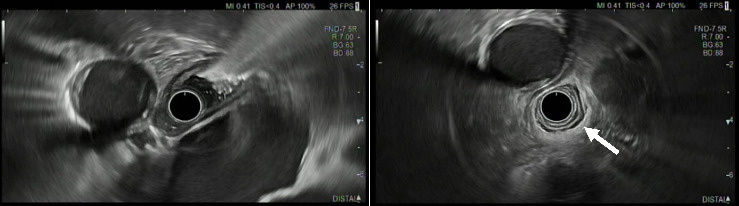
Endosonography images 7th of April 2020, Fujifilm Aloka ARIETTA 850.
